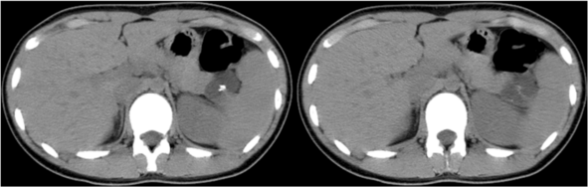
CT scan detected a low-dense and multilocular cystic tumor having thin septum and calcification. The tumor size is 3.3 cm in diameter
